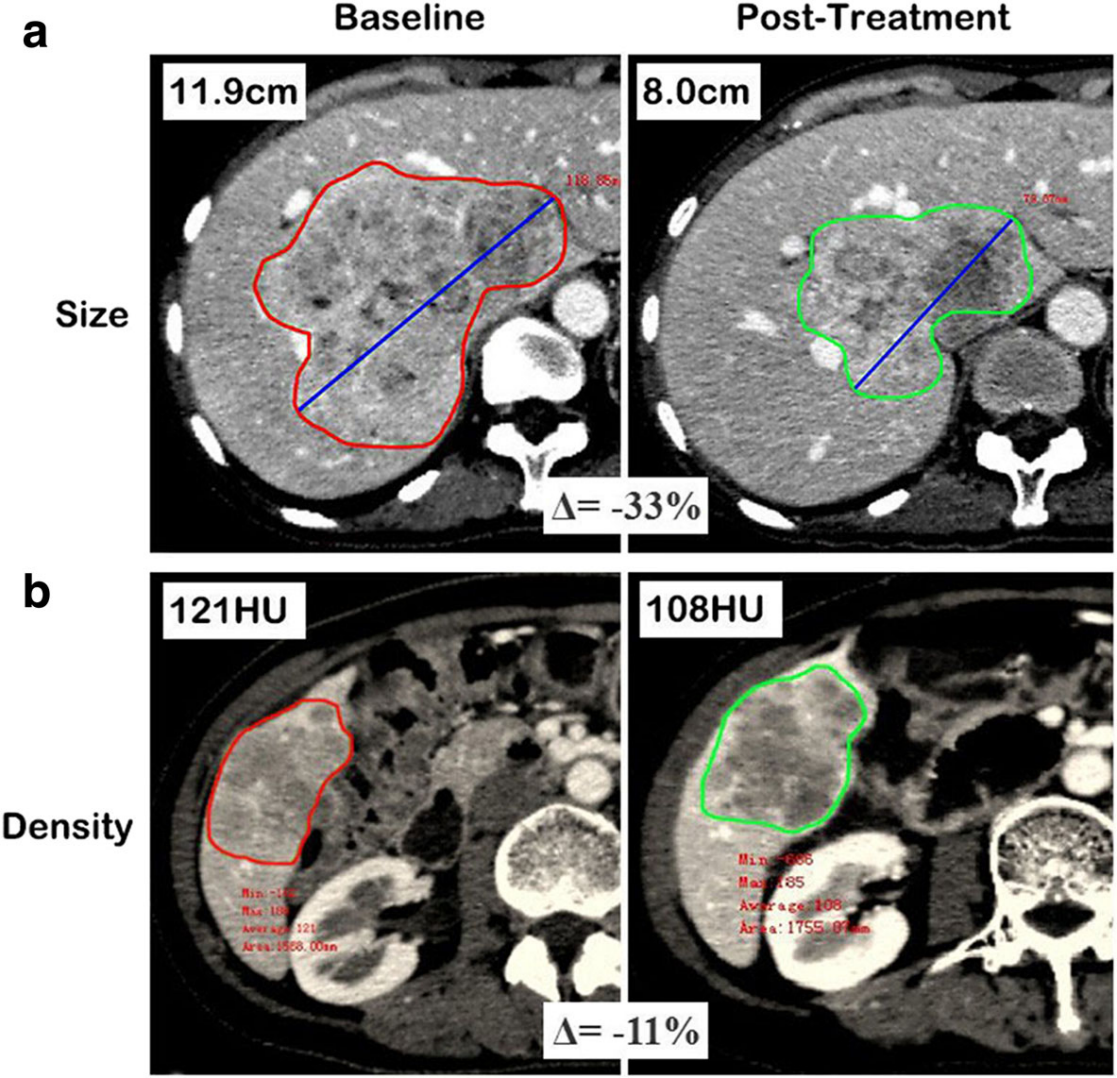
Example of evaluating percentile change in tumor size and density. Pre-treatment and post-treatment CT scans showing shrinkage of hepatic metastases from a pancreatic neuroendocrine tumor in one’s fifties, the percentile change of tumor size was (8.0–11.9)/11.9 × 100% = −33% (a). In another lesion in the same patient, the hepatic metastases appeared more heterogeneous, with high vascularization prior to treatment. Sunitinib induced a large area of tumor hypodensity, suggesting tumor necrosis, the percentile change of tumor attenuation was (108–121)/121 × 100% = −11% (b). In patients with more than one target lesion, the sum of the longest diameters/density of each target lesion in each patient was computed
